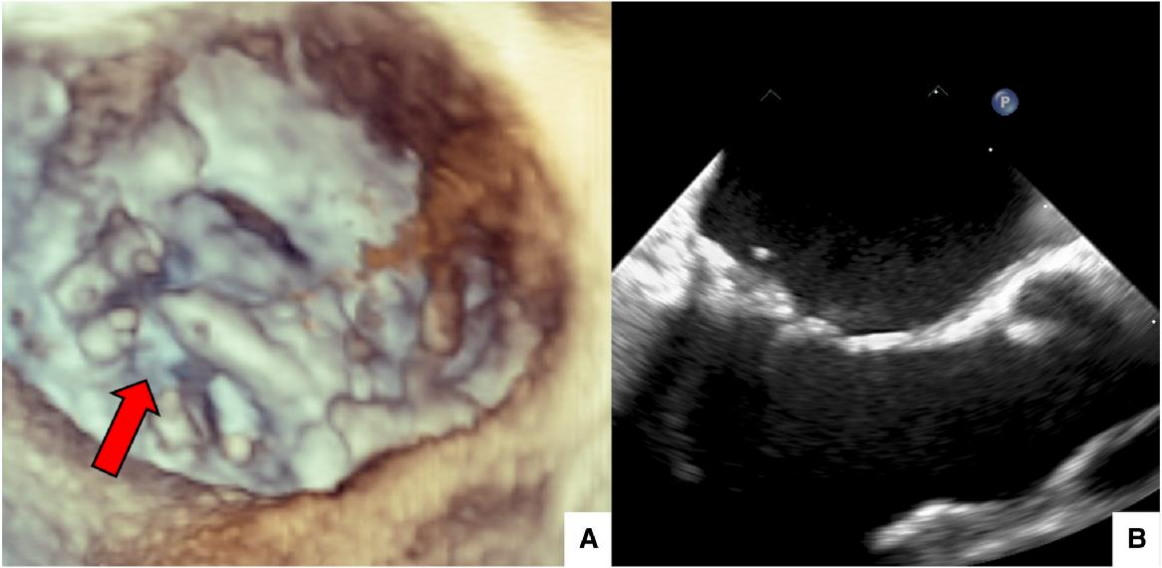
(*A*) Three-dimensional (3D) trans-oesophageal echocardiogram, surgical view. While the medial portion of posterior leaflet’s central scallop (P2M2) is fixed, residual prolapse of its lateral portion (P2M1) persists. (*B*) Two-dimensional (2D) trans-oesophageal echocardiogram, three-chamber view. Systolic mitral annular disjunction and left ventricular infero-basal wall curling have been effectively abolished by annuloplasty.
